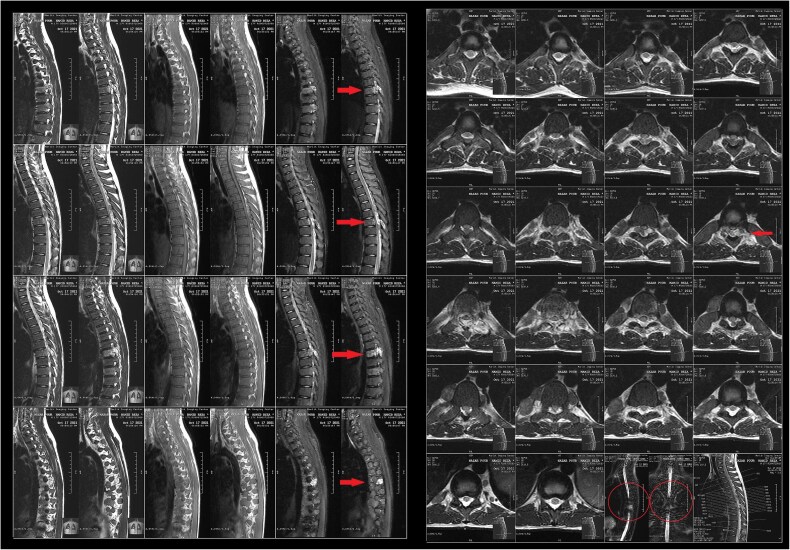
(A) Sagittal and (B) axial T2-weighted MRI images of the dorsal spine, revealing diffuse marrow signal abnormality in the lumbar region, a finding that strongly suggests chronic congenital anemia (thalassemia). Multiple ovoid variable sizes low T1 and T2 signal soft tissue lesions in the right and left of T6, T7, T8, T9, and T9-10 levels, an indicator of extra-medullary hematopoiesis (arrows). A mixed T1 and T2 signal soft tissue in the posterior epidural space at the T6-7, T7, and T8 levels is causing pressure on the thecal sac and cord, leading to canal stenosis at the mentioned levels with edema in the cord (circles), a sign of SCC due to this soft tissue.
